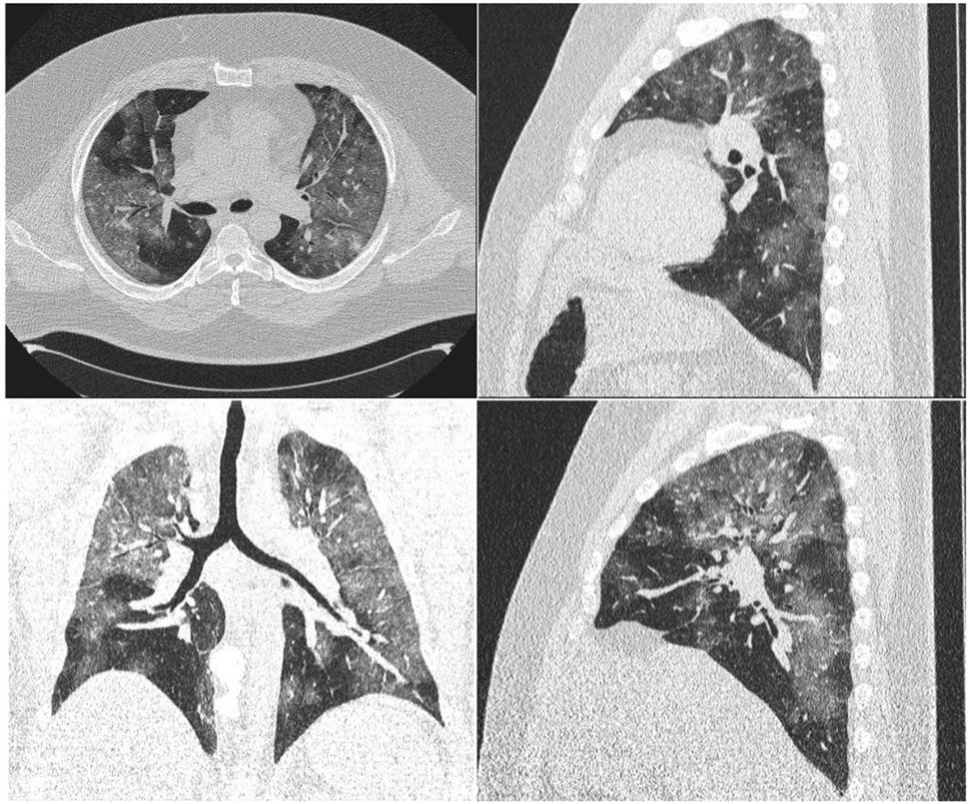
Example of a semiquantitative CT score in a 38-year-old with SarS-CoV-2 pneumonia and a global CT score value of 21
